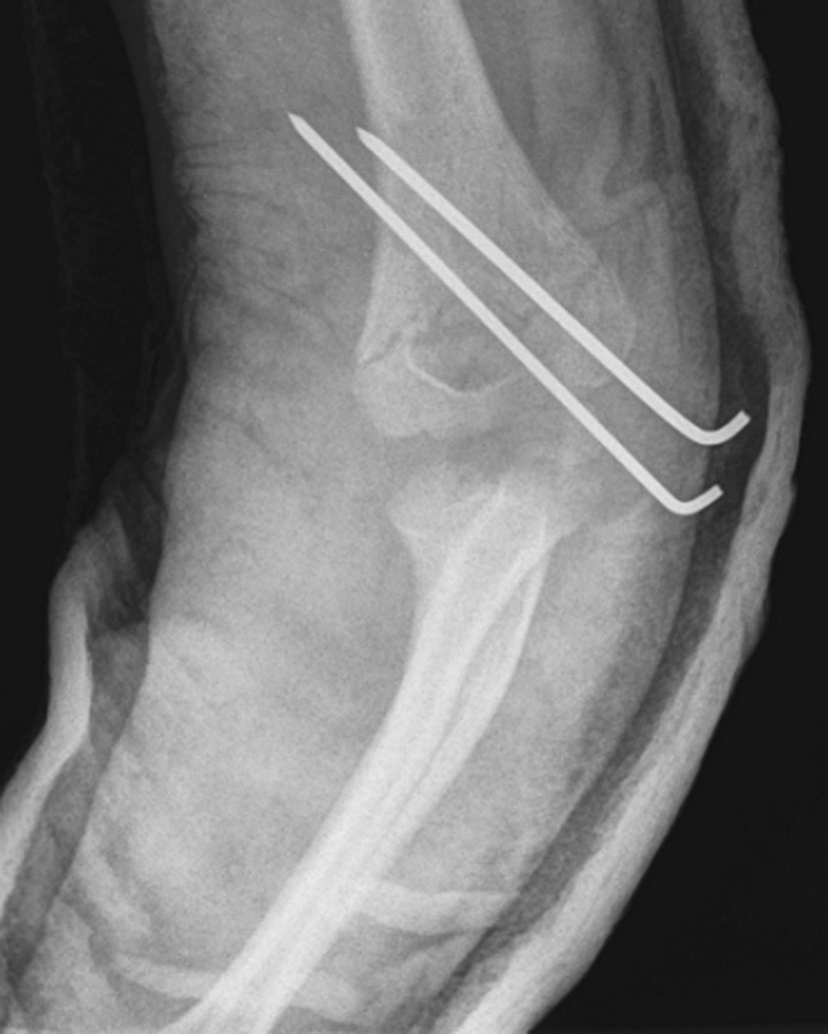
X-ray of third type of supracondylar humerus fracture after reduction—anteroposterior view
